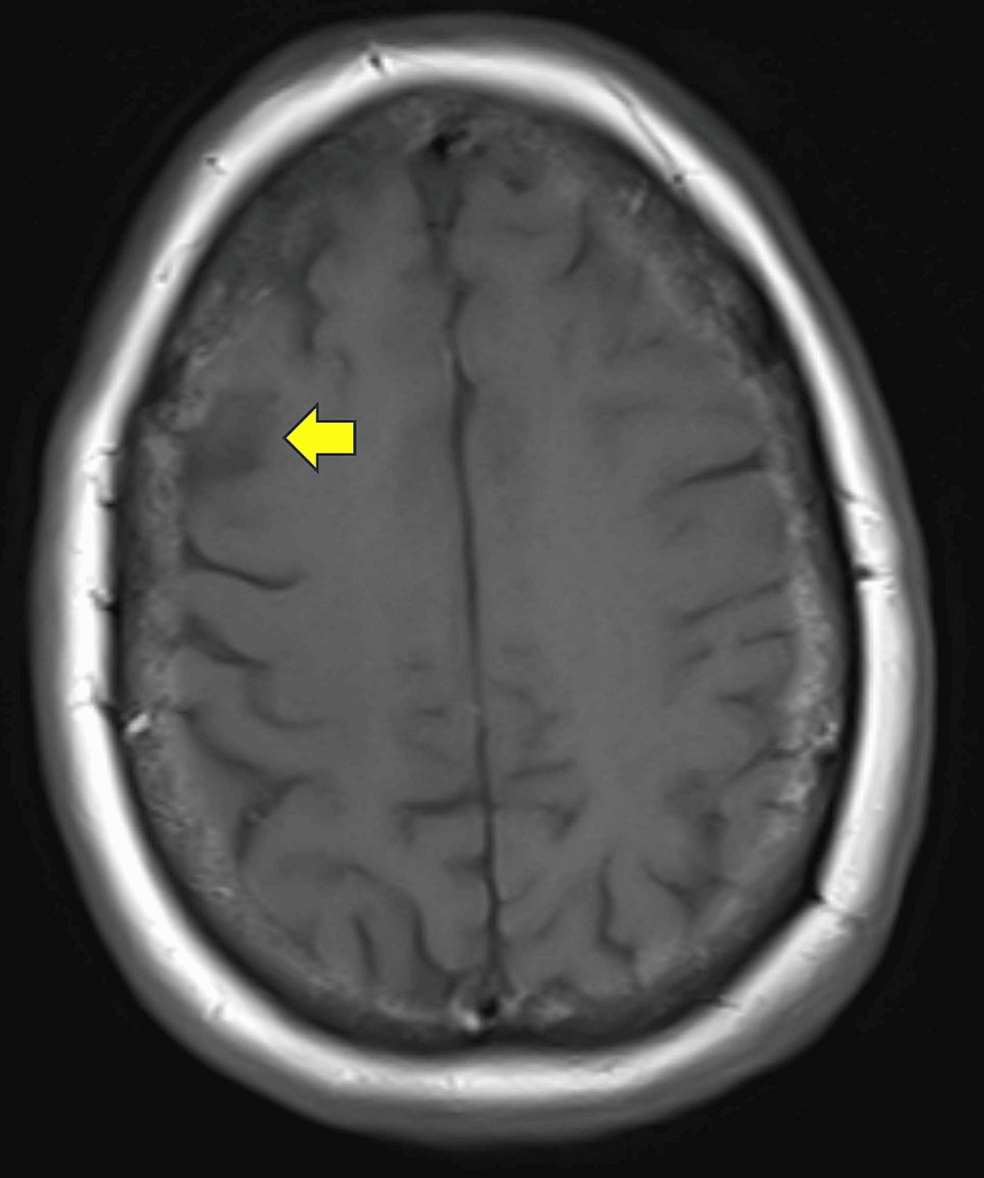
MRI brain without contrast shows subacute ischemia in a right MCA distribution without intracranial hemorrhage Definitions: MRI, magnetic resonance imaging; MCA, middle cerebral artery
